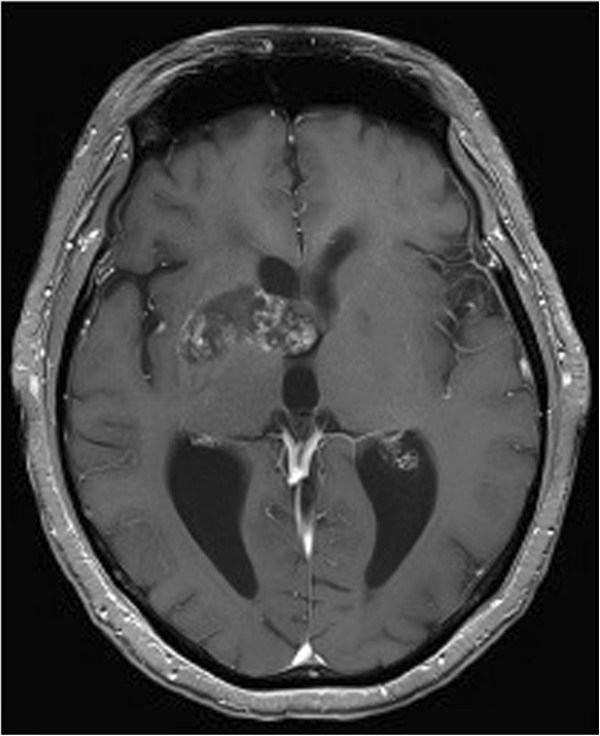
GBM after three courses of bevacizumab. Gadolinium-enhanced T1-weighted MRI shows a reduction in tumor size as well as decreasing and discontinuous tumor enhancement.
